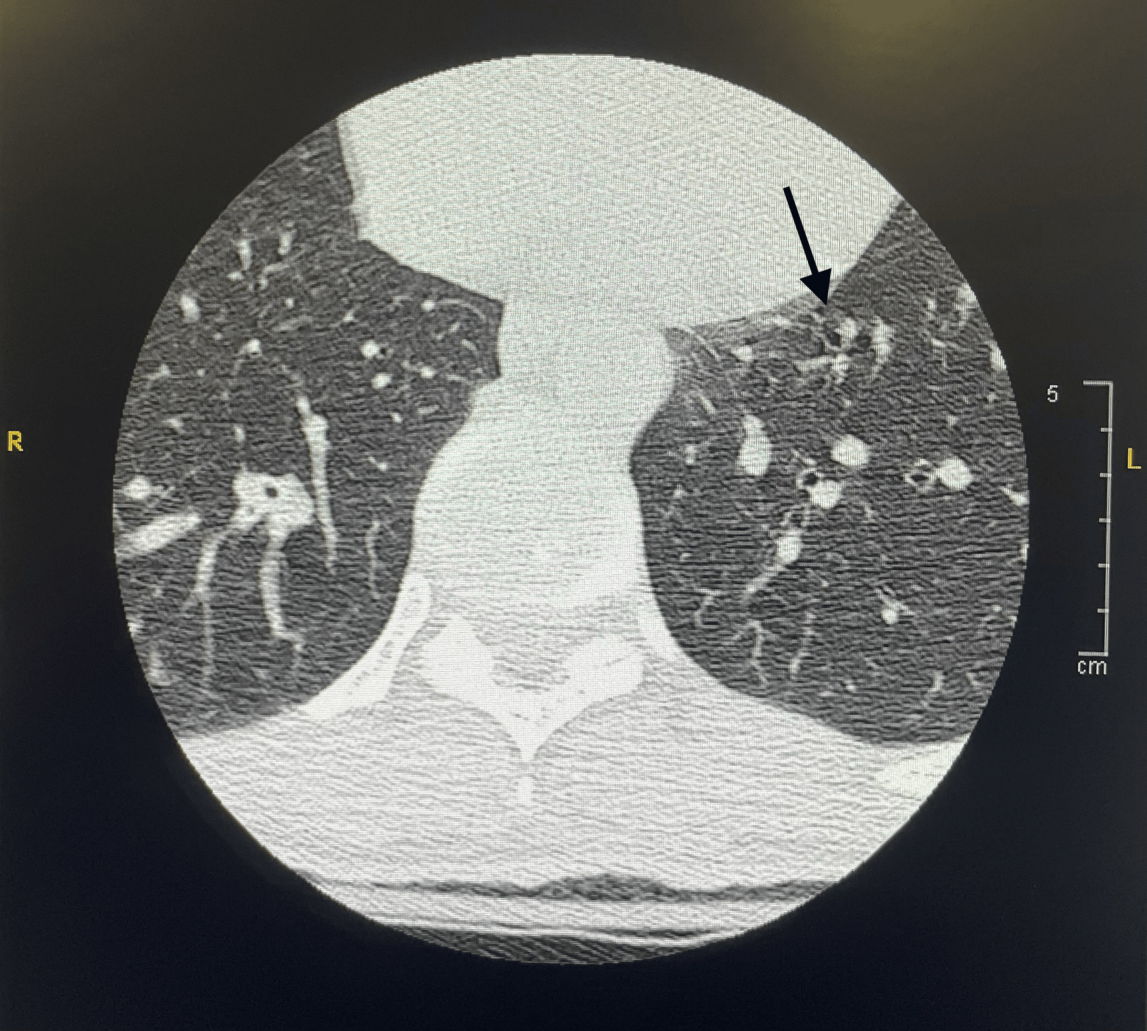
Axial chest CT image demonstrating left lower lobe consolidation (black arrow) with air bronchograms.
